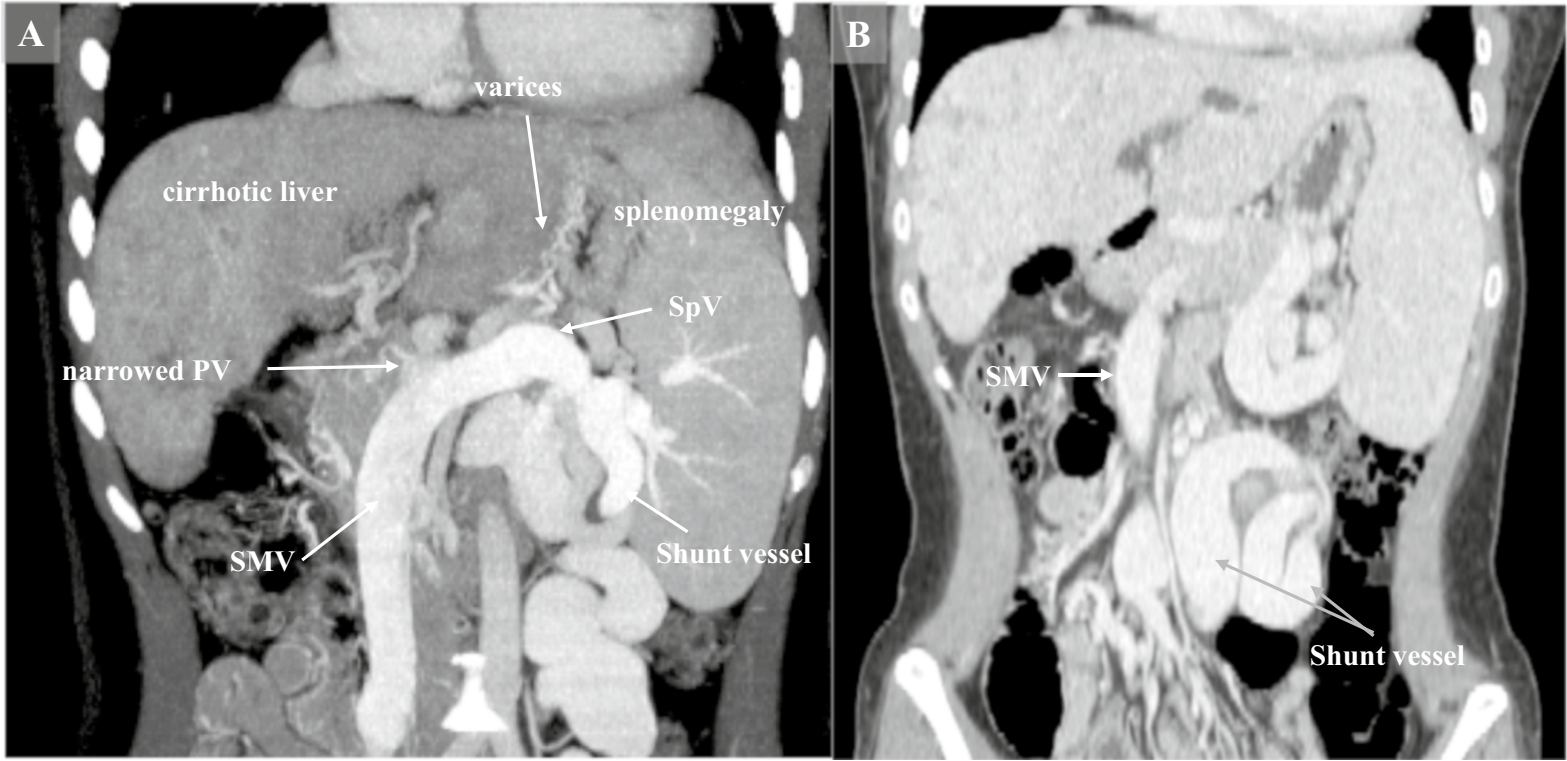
a Preoperative enhanced abdominal CT scans in coronary view showed the hypoplastic portal vein (PV) in 5 mm of diameter and dilated collateral vessels, such as splenic vein, superior mesenteric vein (SMV), and a shunt vessel. The liver exhibited cirrhotic changes accompanied by splenomegaly. b The gonadal vein was increased in length by 20 cm and in thickness by 2 cm and formed the mesosystemic shunt vessel between the SMV and left renal vein
